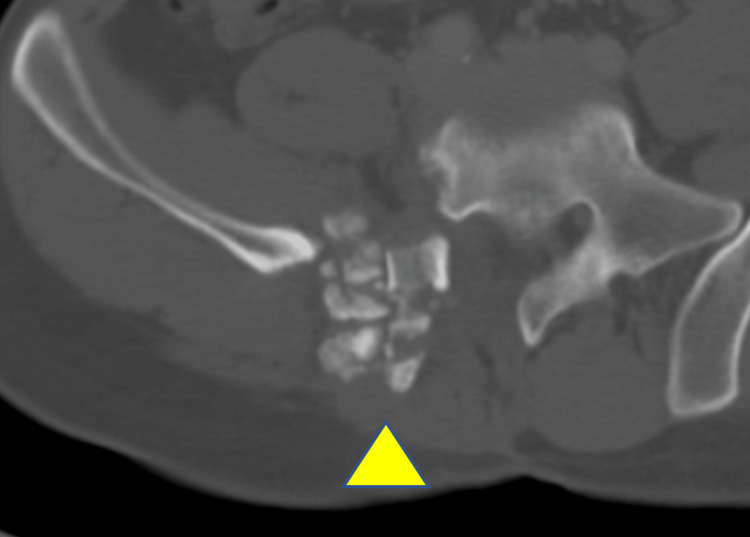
CT imaging after surgery Right sacral wing was resected and the grafted bones were placed (▲) at the same site.
